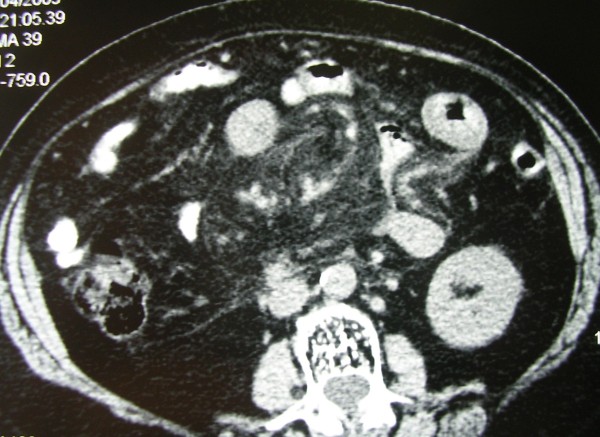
Axial contrast enhanced CT demonstrates a well marginated fat attenuation of the mesentery surrounding the mesenteric vessels. A halo of fat is preserved around the mesenteric vessels and nodules. The lesion is closely related to the adjacent opacified small bowel which is peripherally displaced. The fatty mass is delineated by a hyperdense stripe and accompanied by multiple small nodules.
